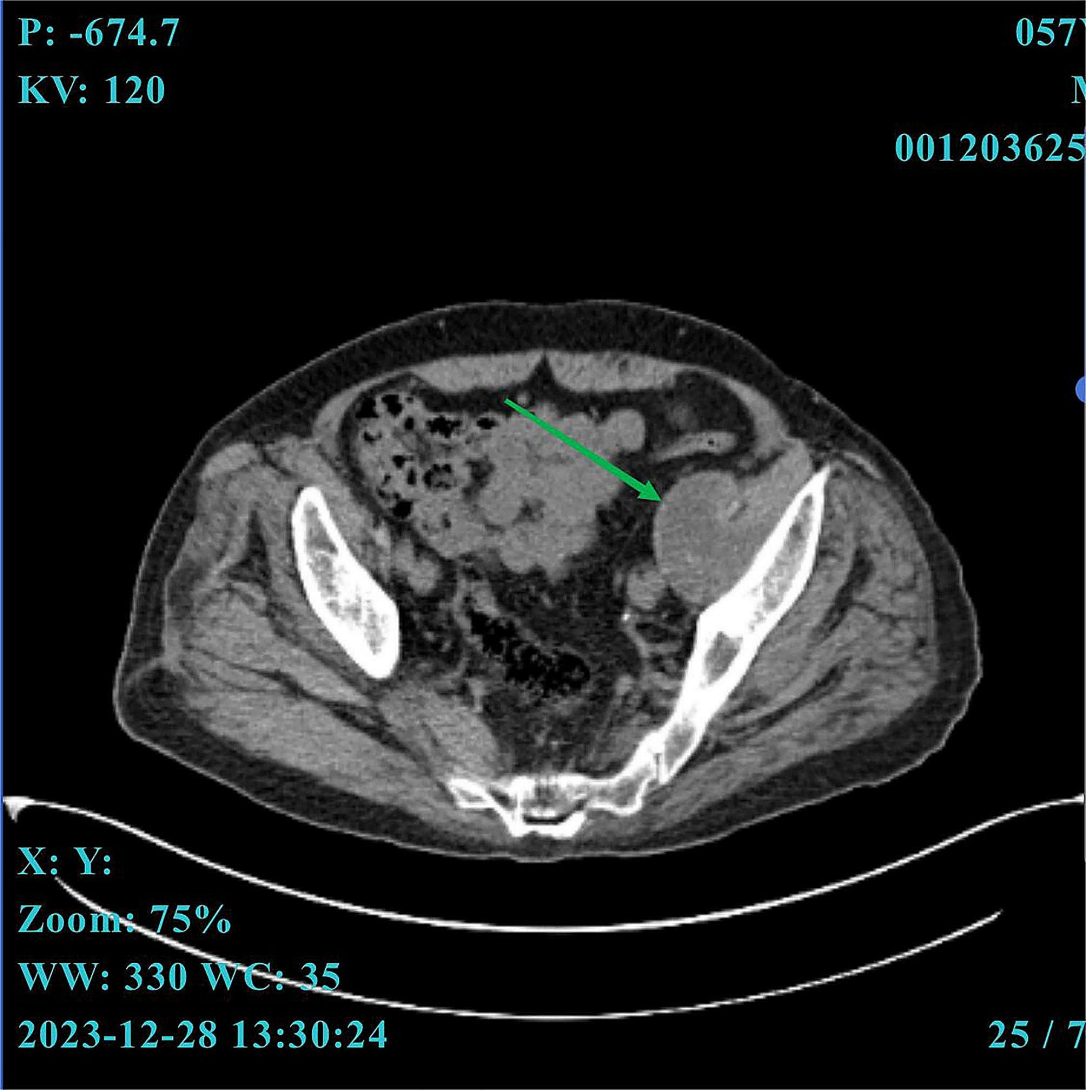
The pelvic CT scan of case 3. An anomalous density mass situated in the left iliopsoas region (green arrows)
